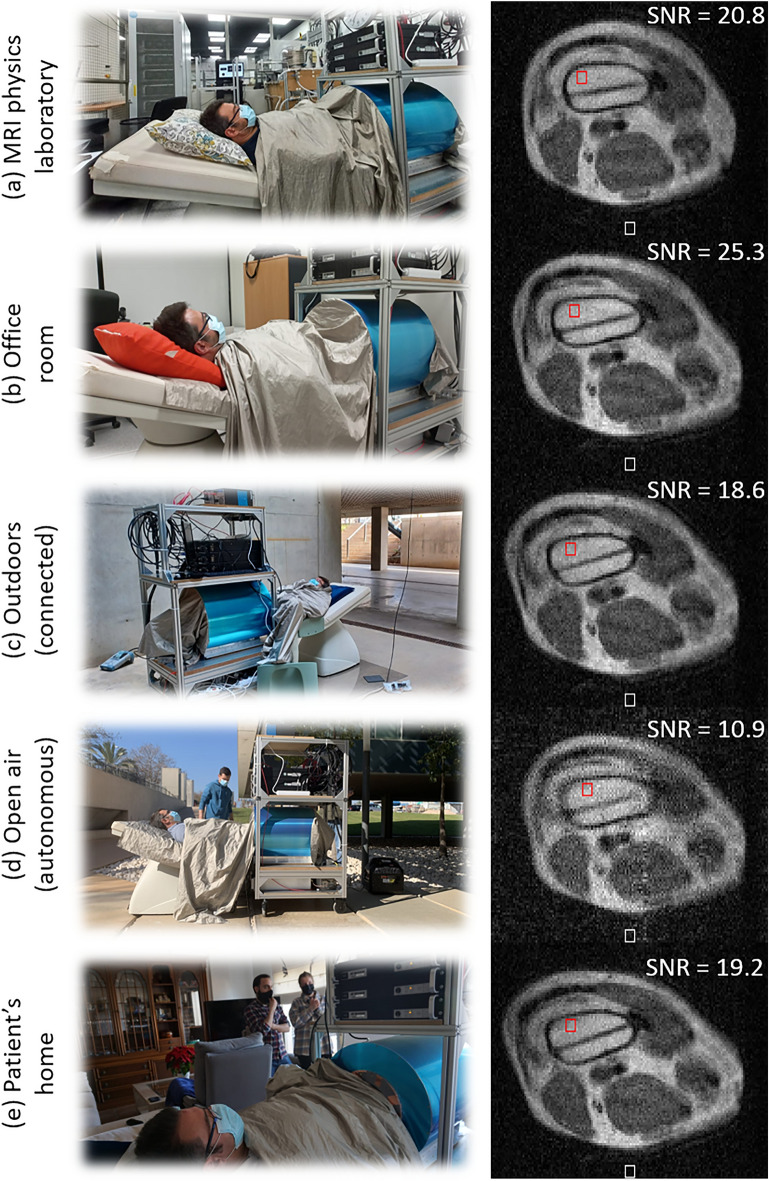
Photographs during acquisitions (left) and axial slice from 3D-RARE reconstructions (right, no post-processing) at five different locations: (a) in an MRI physics laboratory; (b) in an office room; (c) outside a campus building, connected to a nearby power outlet; (d) in open air, powered from a small fuel-based generator; and (e) at the volunteer’s home.
